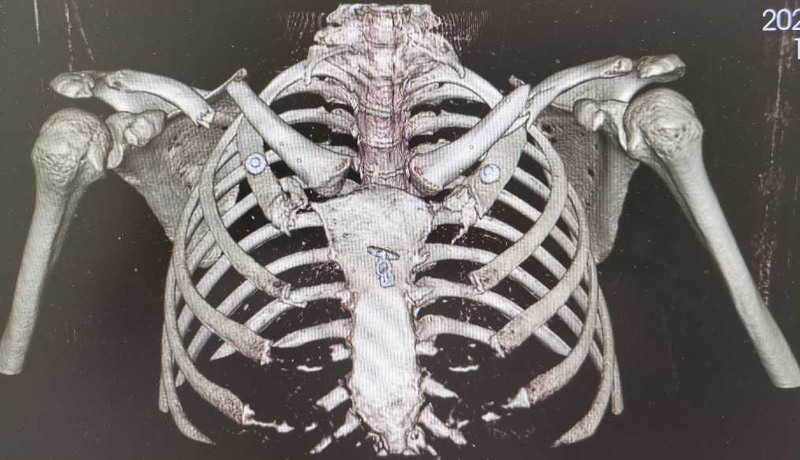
Three-dimensional CT scan on initial presentation: bilateral fractures of the middle clavicle and bilateral incomplete fractures of the 2nd and 3rd ribs. CT = computed tomography.
